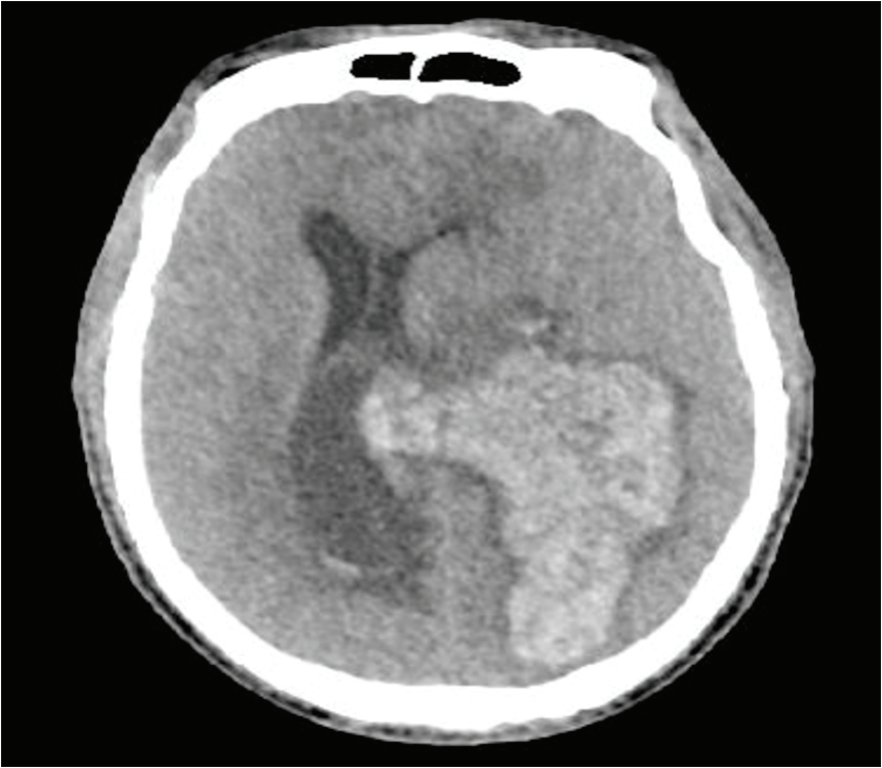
Cranial MRI of the patient after intracranial hemorrhage.
